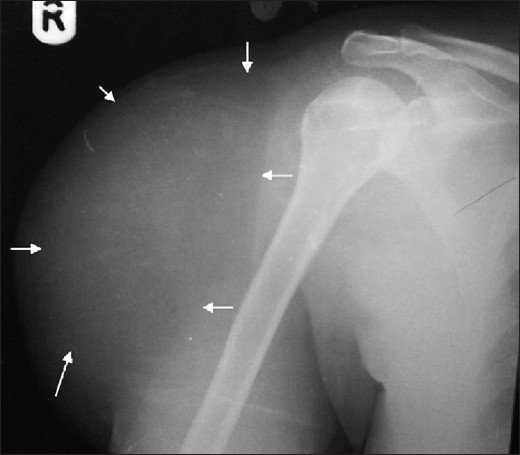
Radiograph showing a subcutaneous lipoma over the right deltoid. Note its well-defined plain (arrow) and the relative radiolucency as compared to the adjacent soft tissue
